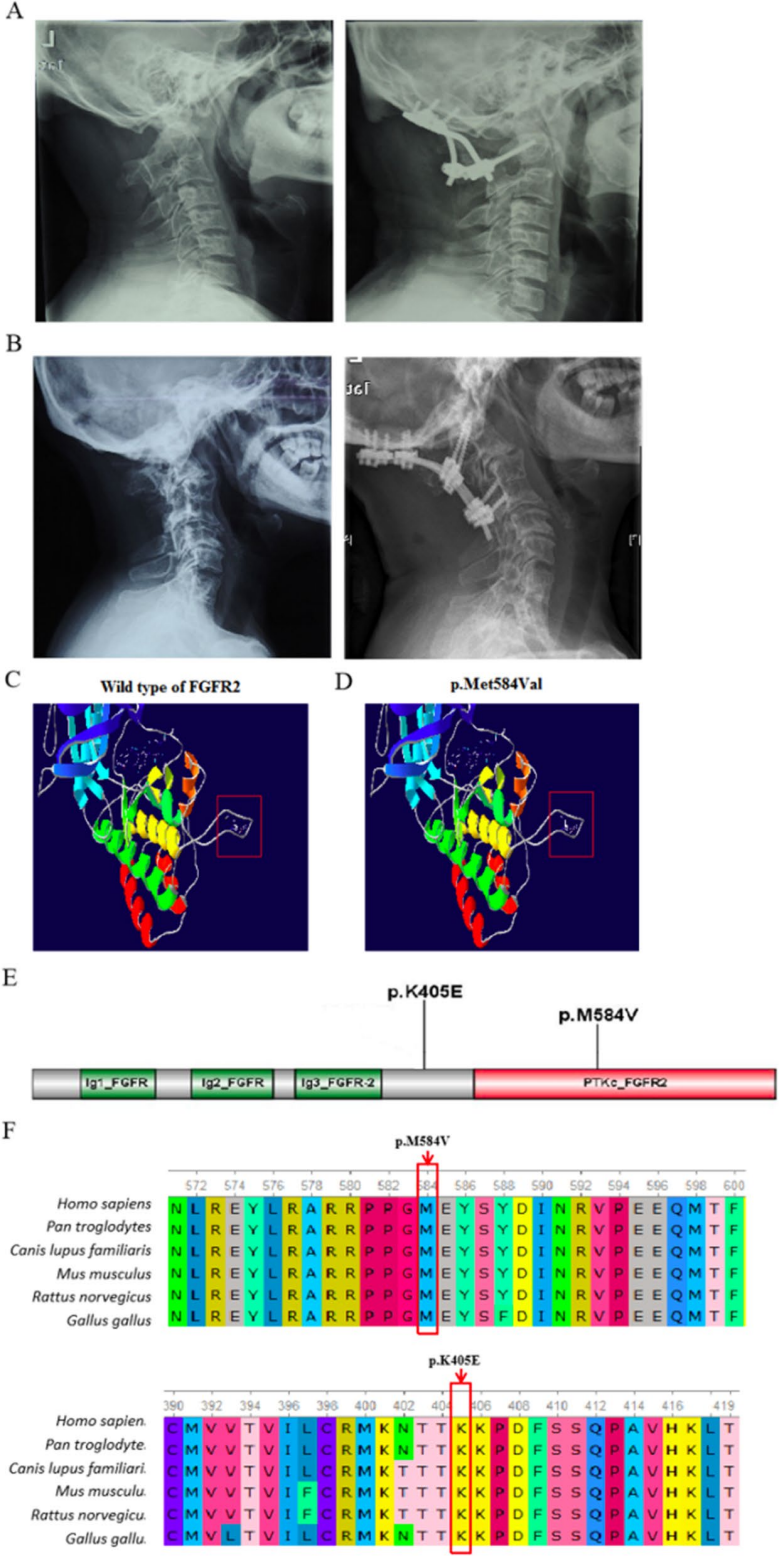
The analysis of variants c.1750A > G (p.M584V) and c.1213A > G (p.K405E) in *FGFR2* in Patients 14 and 9. A, B Pre- and Post-operative lateral X-ray of the cervical spine demonstrating the classic “sandwich deformity” with atlantoaxial dislocation in Patient 14 and Patient 9. C, D SWISSMODEL was used to predict the effects of the mutations in FGFR2 and significant differences between the structure of the mutated and wild-type were identified, indicating structural (red square) and functional change. E Conserved Domain Search was performed, and the variant c.1750A > G (p.M584V) was located in the PTKc domain of the protein. F Multiple sequence alignments revealed that the mutation sites were highly evolutionarily conserved
